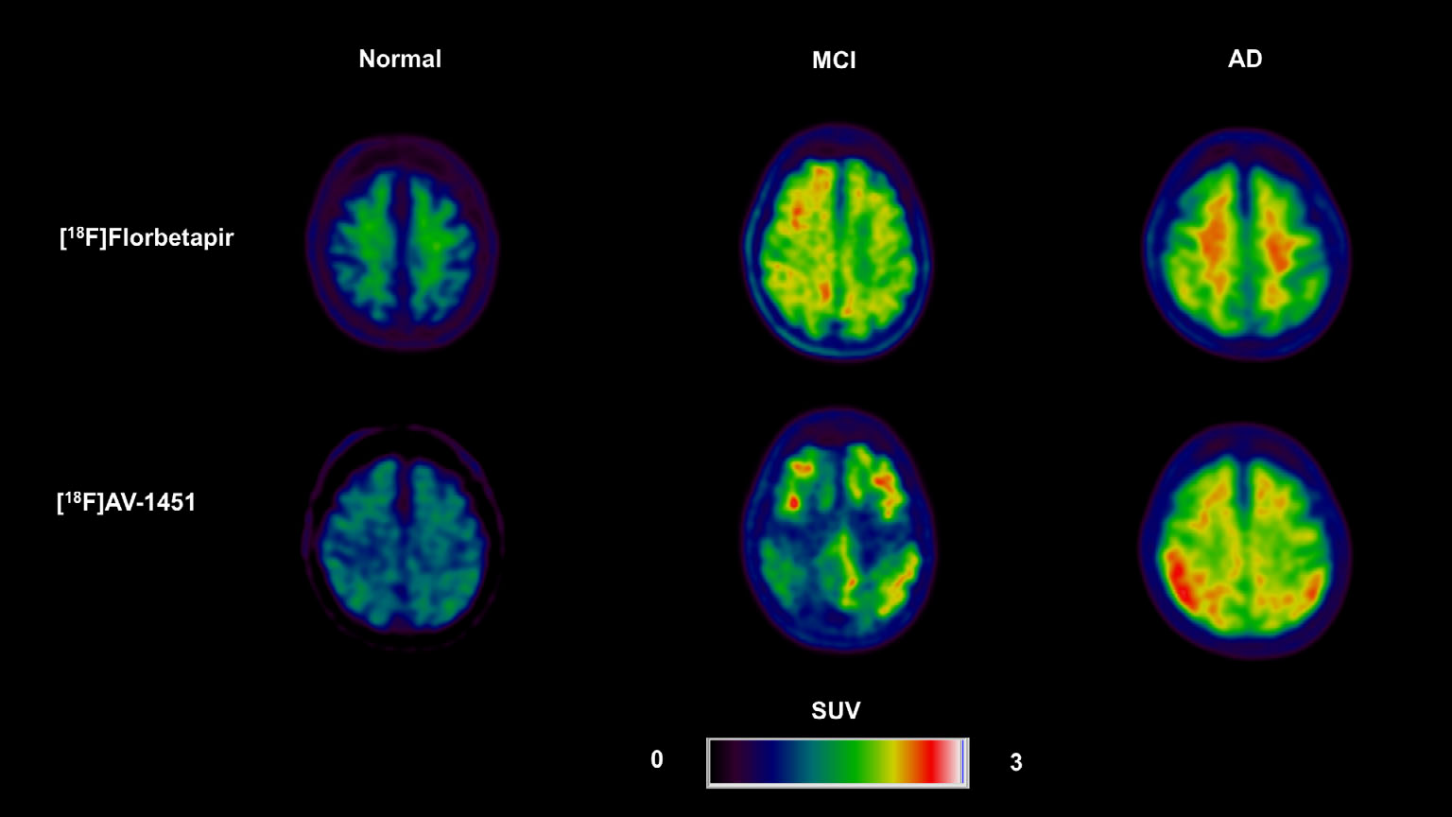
Positron emission tomography (PET) imaging as quantified by standardised uptake values (SUVs) depicting increased tracer uptake for [^18^F]florbetapir and [^18^F]AV‐1451 in an Alzheimer's disease (AD) patient and a mild cognitive impairment (MCI) patient compared to a healthy control [Color figure can be viewed at http://wileyonlinelibrary.com]
